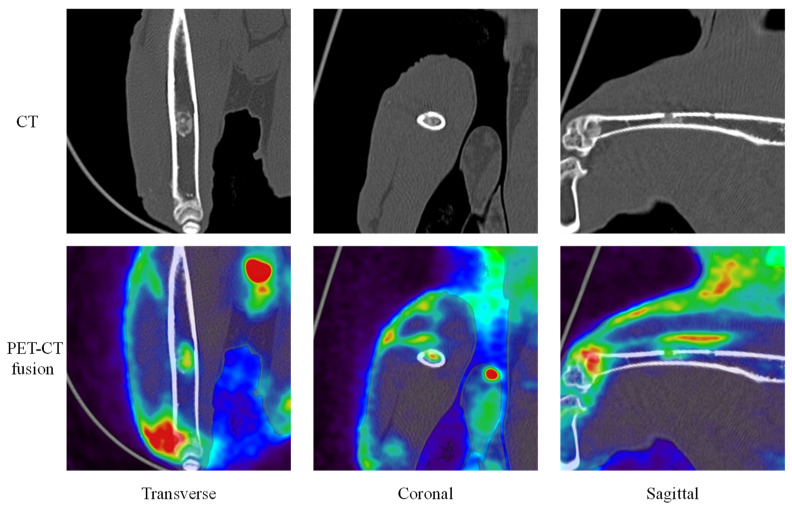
Example of fusion of CT data and PET images. With Transverse, Coronal and Sagittal views, the PET images are superimposed on the CT image in all directions. As probe accumulation increases, the display color in the PET images shifts from green to yellow and subsequently to red.
